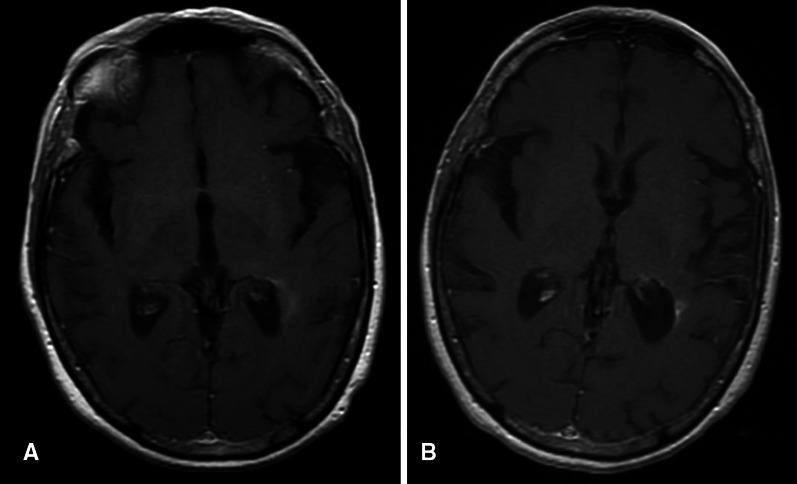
MRI demonstrating a durable response. The patient had a biopsy and treatment of GB anterior and caudal to this lesion. The enhancing area in A appeared and progressed while the patient was receiving BEV, almost a year and a half after first line therapy. The response occurred slowly, over months, while the patient was receiving EP. a T1-post contrast at time of progression on BEV, b T1-post contrast at approximately 45 weeks on EP
